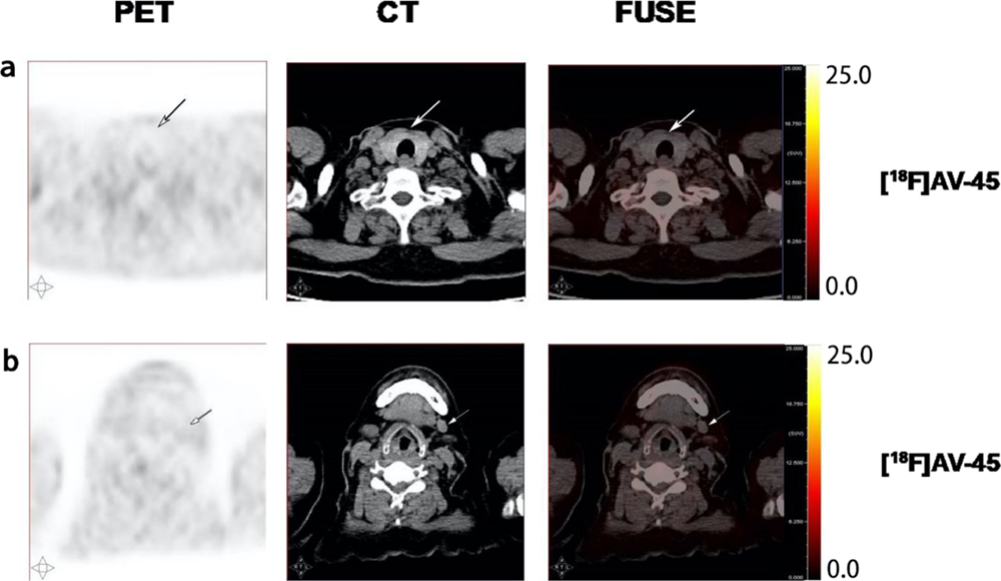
[^18^F]AV-45 PET/CT images of a healthy human subject.
